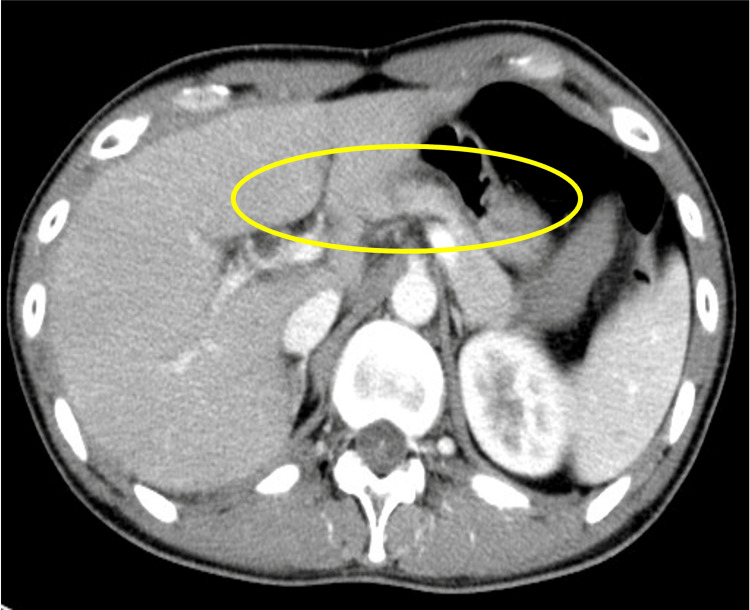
Contrast-enhanced CT scan of the abdomen Axial view of contrast-enhanced abdominal CT. The pancreas is unremarkable, and no abnormal findings are observed in surrounding retroperitoneal structures.
